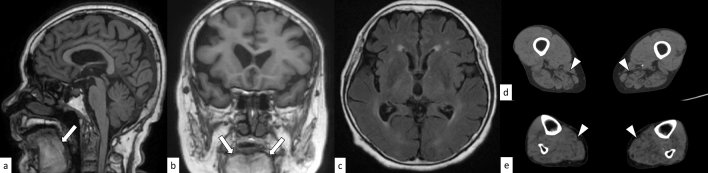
A 76-year-old woman with OPMD. T1-weighted sagittal image a and coronal image b show fat infiltration of the tongue (arrows). No significant abnormal finding is found in the brain (c: FLAIR axial image). Atrophy of the bilateral extensor muscles in the lower extremities is observed on non-enhanced CT (d, e, arrowheads)
